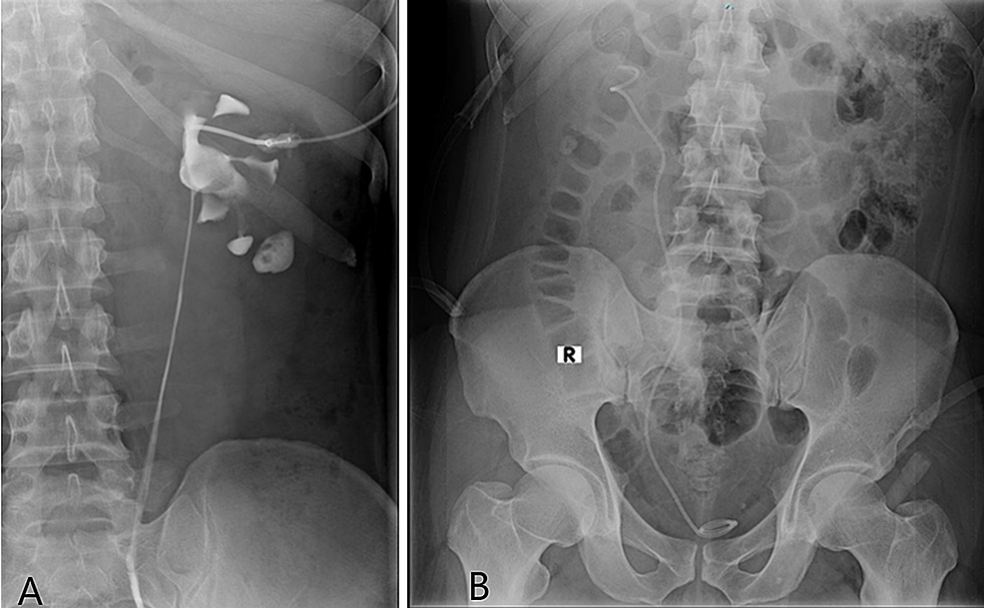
Image showing the CT urogram and kidney, ureter, and bladder X-ray. (A) Postop urogram showing the patient double-J stent in the upper calyx and patient ureter. (B) Postop kidney, ureter, and bladder X-ray showing DJ stent placement.
